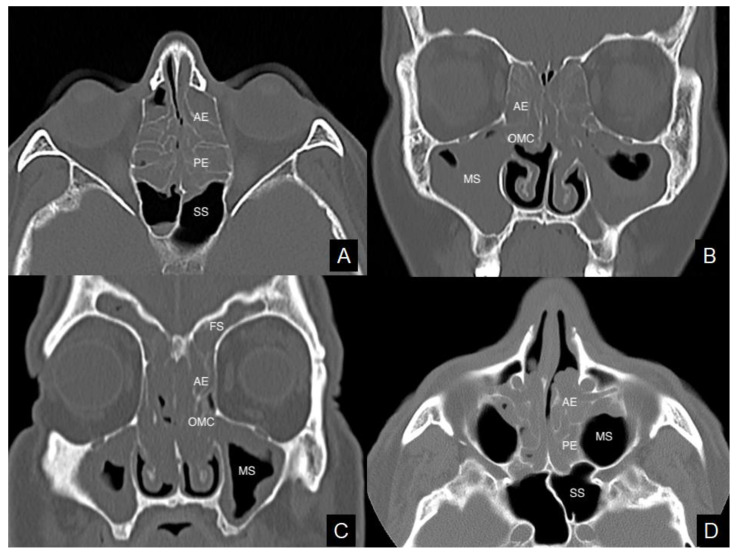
Axial and coronal views of computed tomography imaging. Massive and homogeneous sinus opacification in a chronic rhinosinusitis with nasal polyps (CRSwNPs) patient with non-steroidal anti-inflammatory drugs-exacerbated respiratory disease (NERD) (A,B). Ethmoid inflammatory involvement in a CRSwNPs case with asthma (C). Polyps occupying the central compartments in a patient with eosinophil CRSwNPs (D). AE = anterior ethmoid; MS = maxillary sinus; OMC = ostiomeatal complex; PE = posterior ethmoid; SS = sphenoidal sinus. FS = frontal sinus.
